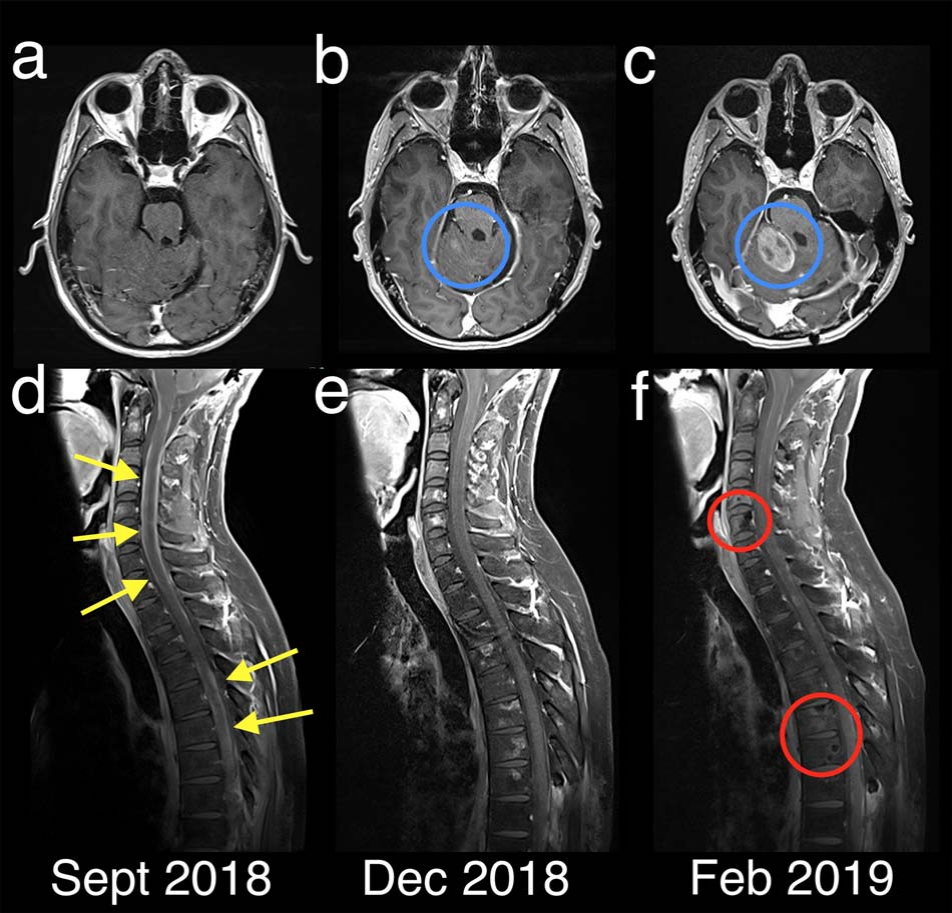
MRI brain and whole spine with Axial T1 post-contrast (a–c) and sagittal T1 post-contrast FS (d–f) following commencement of Sonidegib. In September 2018 (a/c) there was evidence of severe leptomeningeal spread along the spinal cord (yellow arrows) which initially improved on Sonidegib therapy. However, over time there was marked progression of intracranial disease (blue circles) and increased bony metastatic disease (red circles) which preceded further intracranial leptomeningeal spread prior to transition to palliative care.
